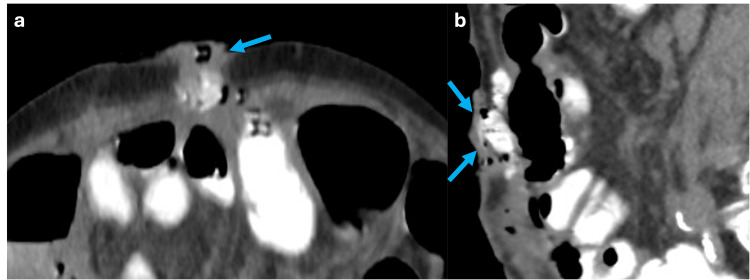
Axial and sagittal abdominal CT scan with oral contrast showing an enteroatmospheric fistula (a, b) Axial and sagittal CT scan shows a fistulous tract communicating the small bowel with the abdominal wall in the umbilical region, consistent with an enteroatmospheric fistula (blue arrows). The figure was created by the authors.
